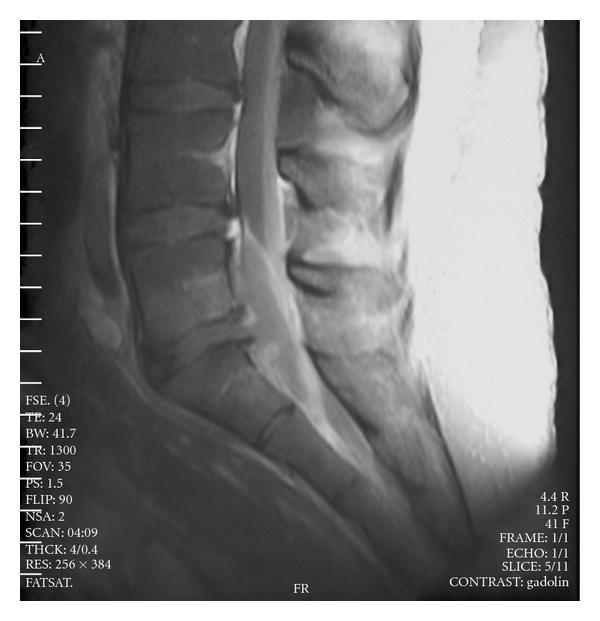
Sagittal T2-weighted, gadolinium-enhanced NMR imaging demonstrates enhancing *epidural collection* ventrally compressing the thecal sac opposite the L5-S2 vertebrae. The L5-S1 disk space is partially obliterated and the bony end plates enhanced, reflecting *discitis and osteomyelitis*.
